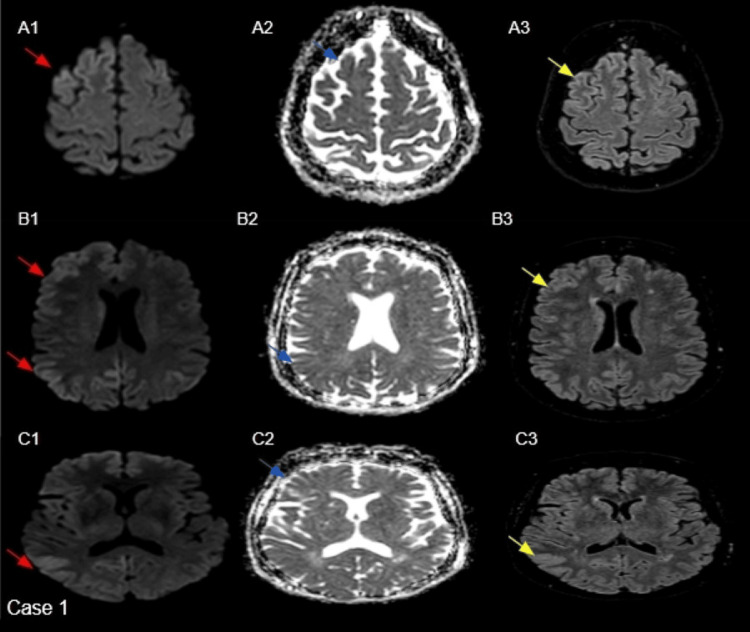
Axial B-1000 diffusion images (red arrows in A1, B1, and C1) demonstrate cortical hyperintensity involving the bilateral frontoparietal convexities, more pronounced on the right. Corresponding ADC maps (blue arrows in A2, B2, and C2) show mildly reduced signal in the same regions, and FLAIR images (yellow arrows in A3, B3, and C3) reveal subtle hyperintensity. The basal ganglia, thalami, and cerebellum appear normal. ADC: apparent diffusion coefficient; FLAIR: fluid-attenuated inversion recovery
